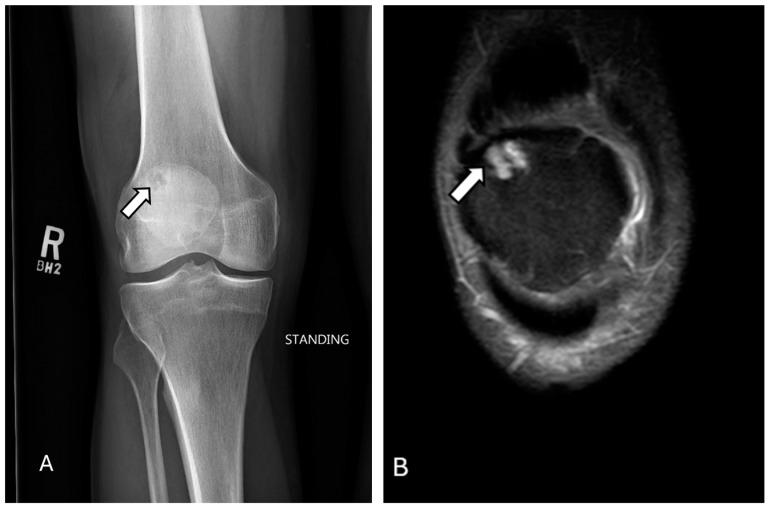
Dorsal defect of patella. Anterior–posterior knee X-ray (A) and coronal proton density fat-suppressed image (B) show a well-marginated 12 mm lesion (arrows) at the superolateral aspect of the dorsal patella with no associated marrow edema, most likely representing a dorsal patellar defect. The images were obtained from the University of Washington Medical Imaging database with the required permissions.
